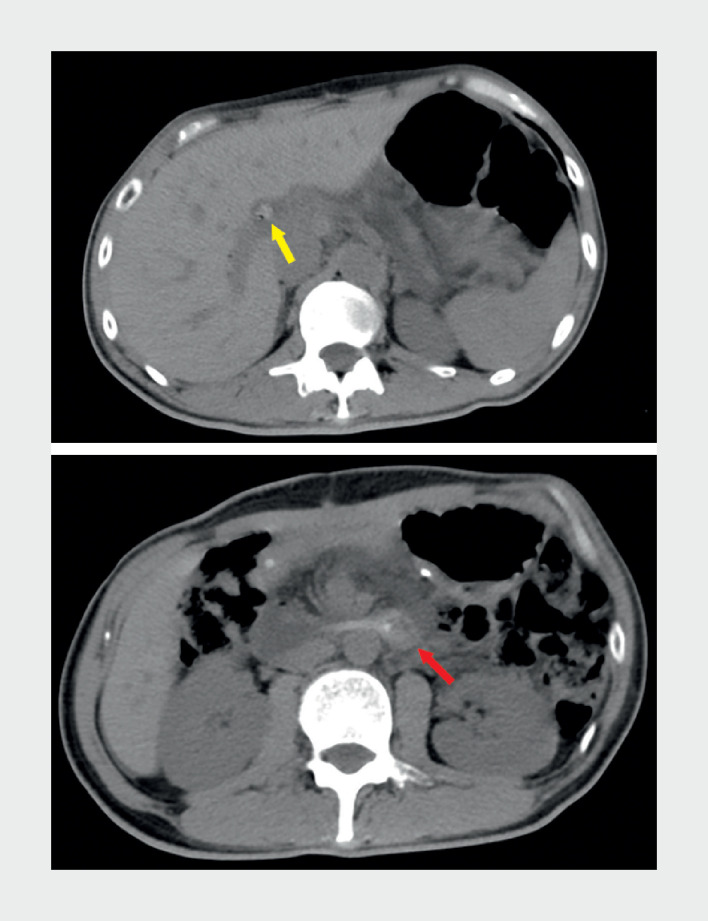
Abdominal computed tomography scans revealed high-density images at the terminus of the pancreatic stent tube (red arrow) and the hilar bile duct (yellow arrow).
